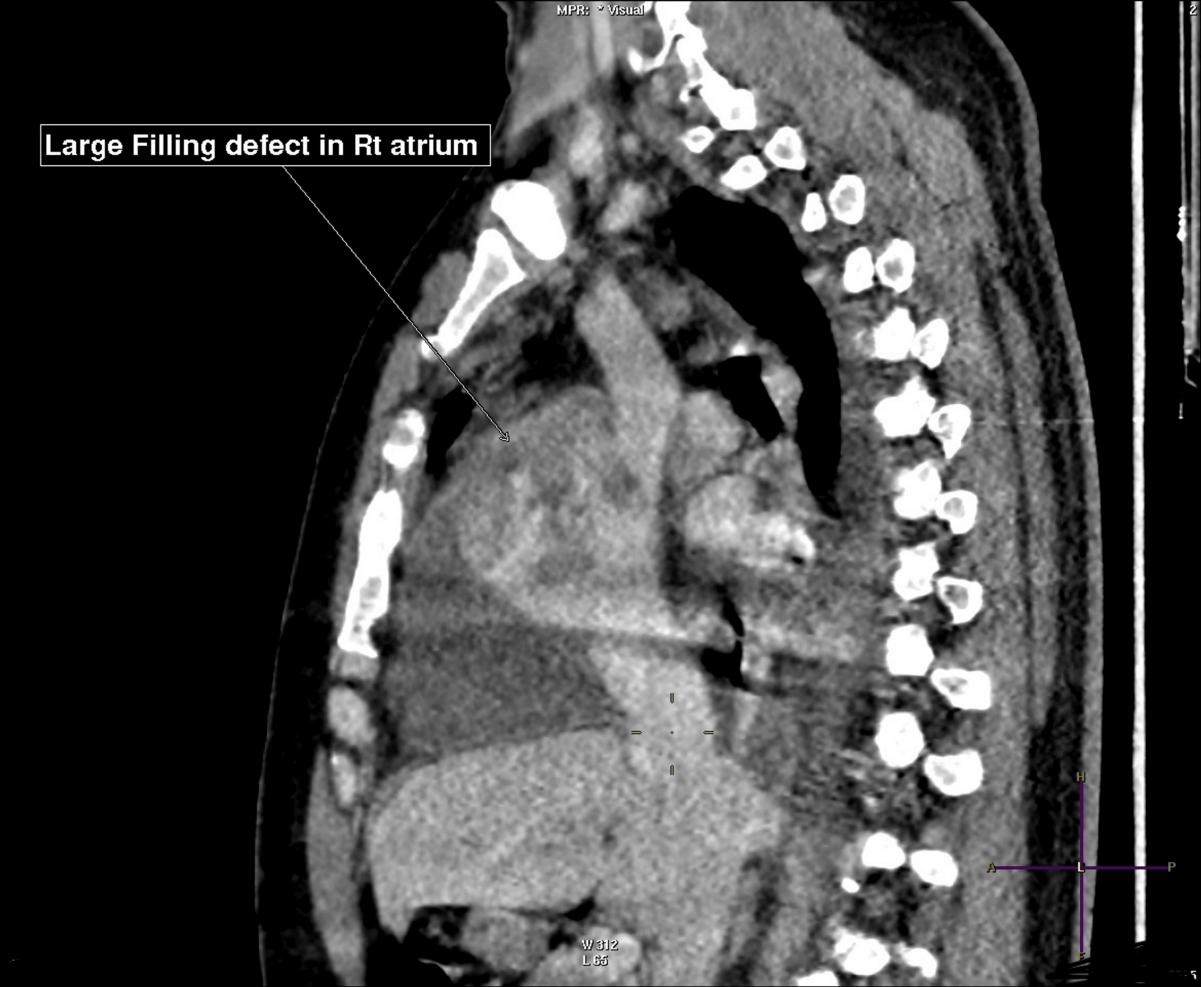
Computed tomography (CT) chest showing right atrial mass extending to the superior vena cava
